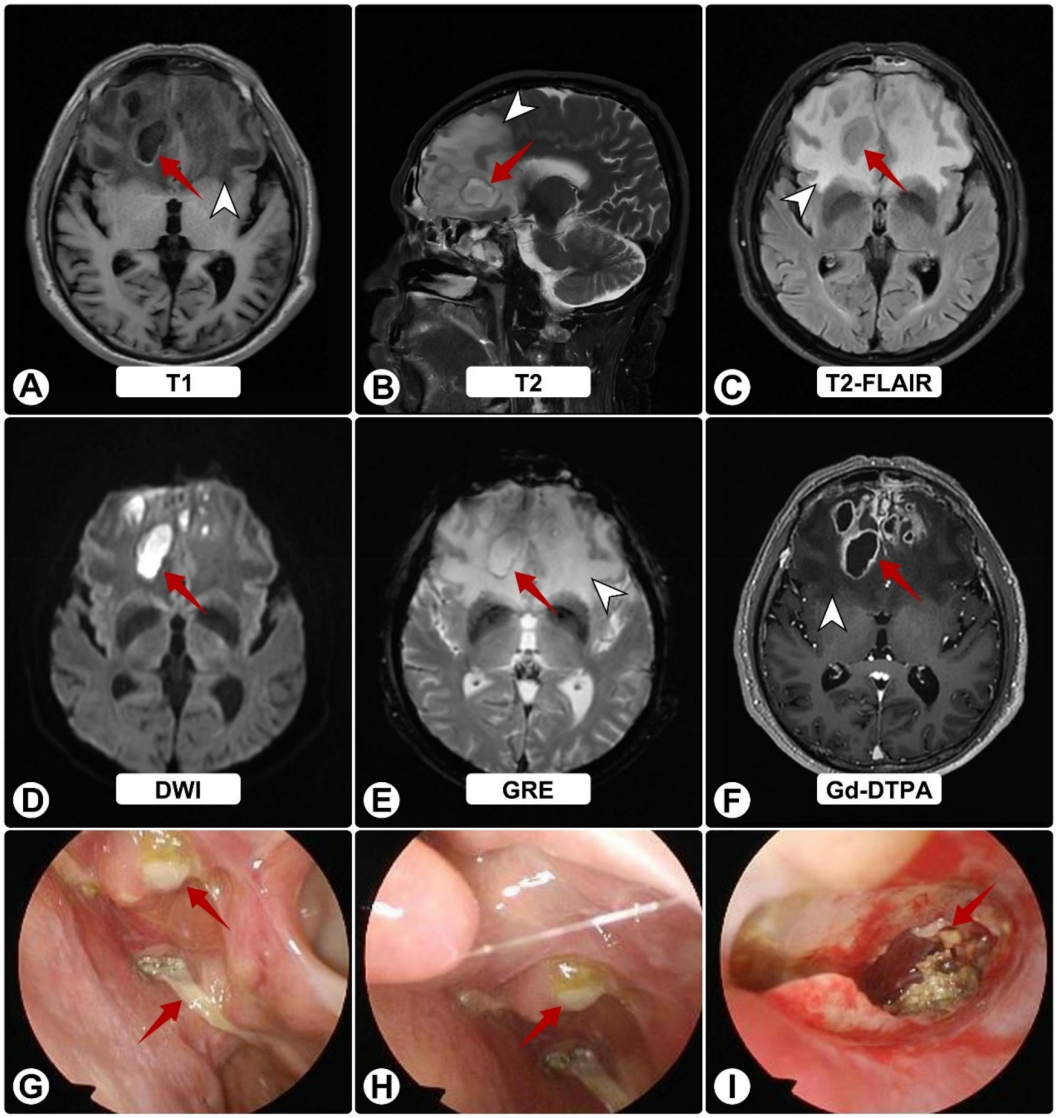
Brain MRI and nasal endoscopy on admission. (A–F) White arrowheads indicate surrounding edema; red arrows show abscesses. MRI shows bilateral frontal lesions appearing hyperintense on T1 (A) and T2 (B), and isointense on FLAIR (C) Post-contrast images (D–F) reveal ring enhancement of lesions with extensive vasogenic edema and midline shift. (G–I) Nasal endoscopy shows purulent sinus secretions (red arrows).
